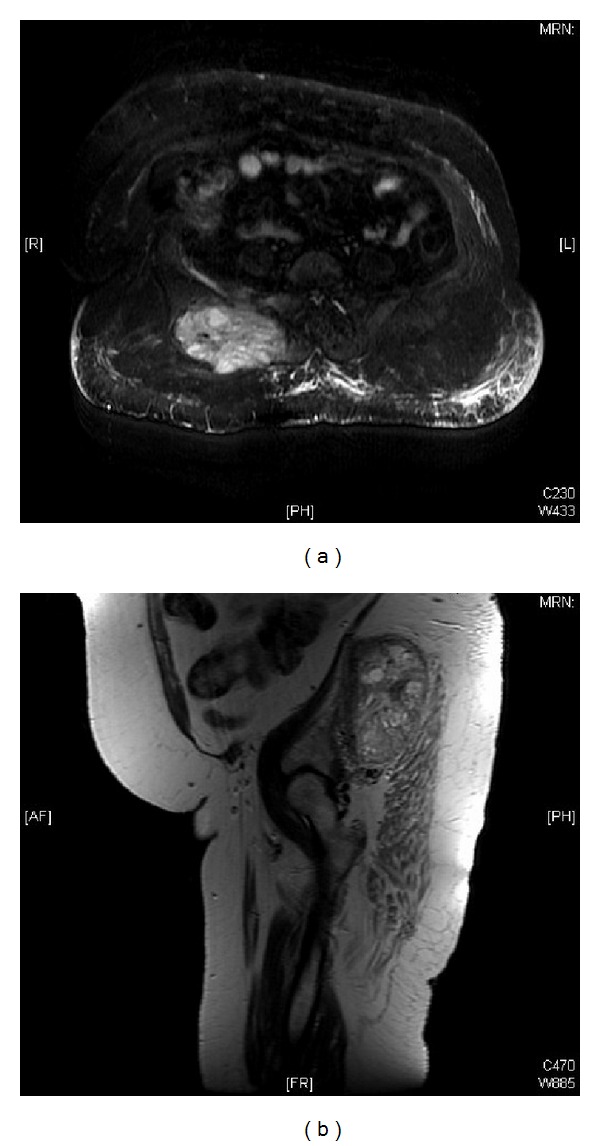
Magnetic resonance imaging (MRI) of the pelvis showing 13 × 11.7 × 6.8 cm lobulated heterogeneous mass in the right gluteus medius muscle also involving the right gluteus maximus, piriformis muscles extending to the right iliac bone and the right sacroiliac joint.
